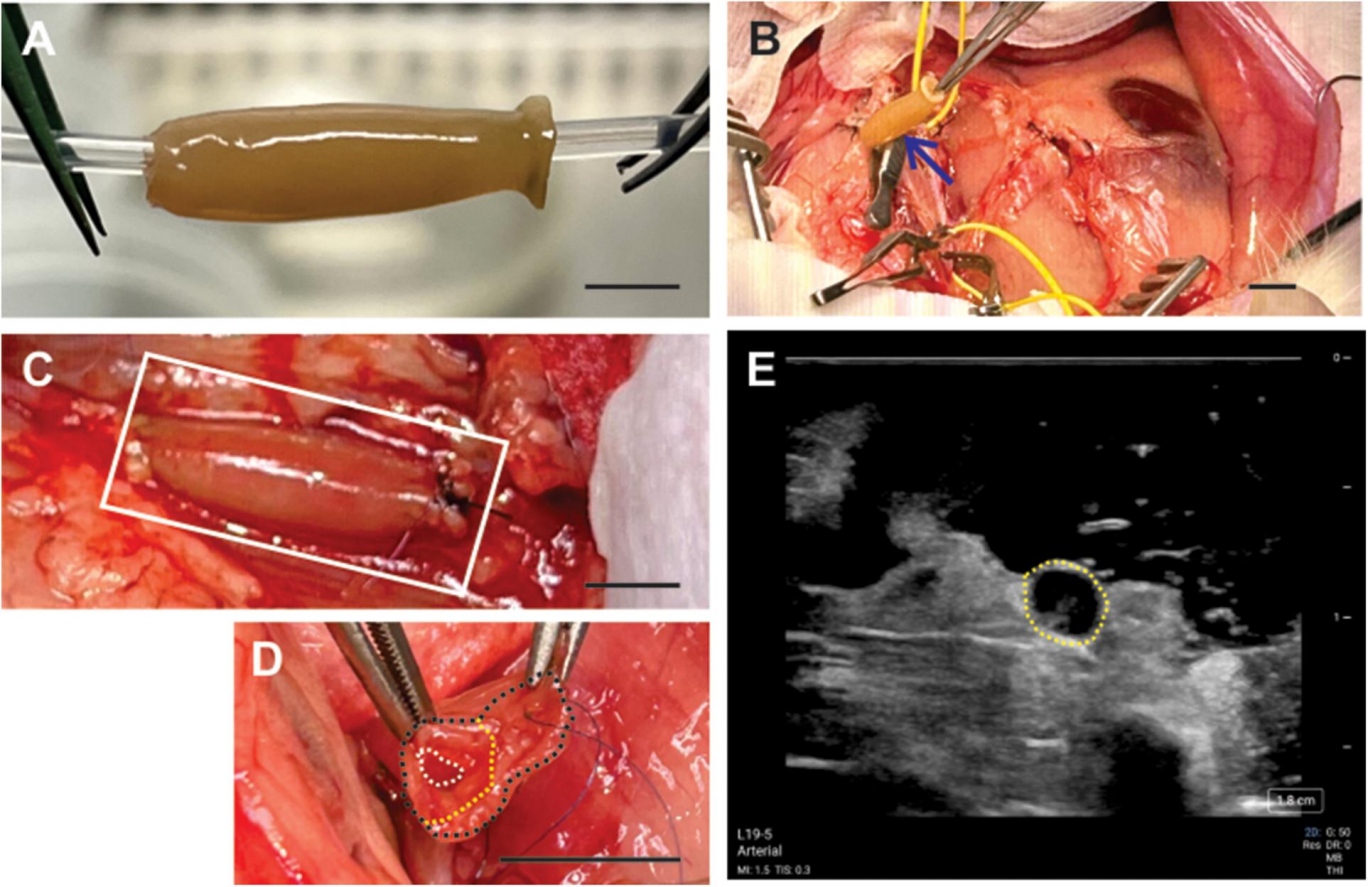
All-natural tissue engineered vascular graft implanted into a rabbit abdominal aorta model. (A) Engineered graft built in the laboratory composed of human cells; total length was 2.2 cm. (B) Surgical site of the graft (blue arrow). (C) Anastomosed graft (white box) to the abdominal aorta. (D) Graft dissected between its two layers following blood perfusion, marked by the outer vessel wall (black dashed line), inner lumen (white dashed line), and the dissected region (yellow dashed line). (E) Ultrasound showed vessel dissection within the vessel lumen (yellow dashed line). Scale bars = 1 cm.
